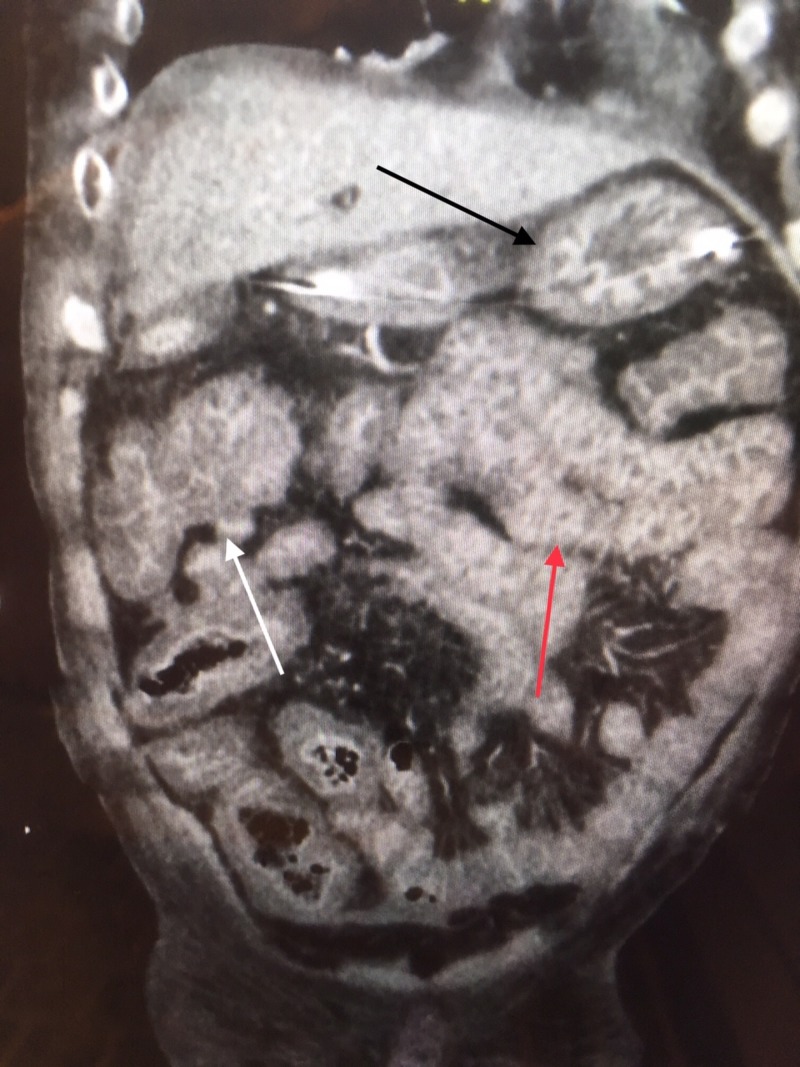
CT abdomen and pelvis Black arrow: inflamed stomach; white arrow: inflamed colon; red arrow: inflamed small bowel CT: computed tomography
